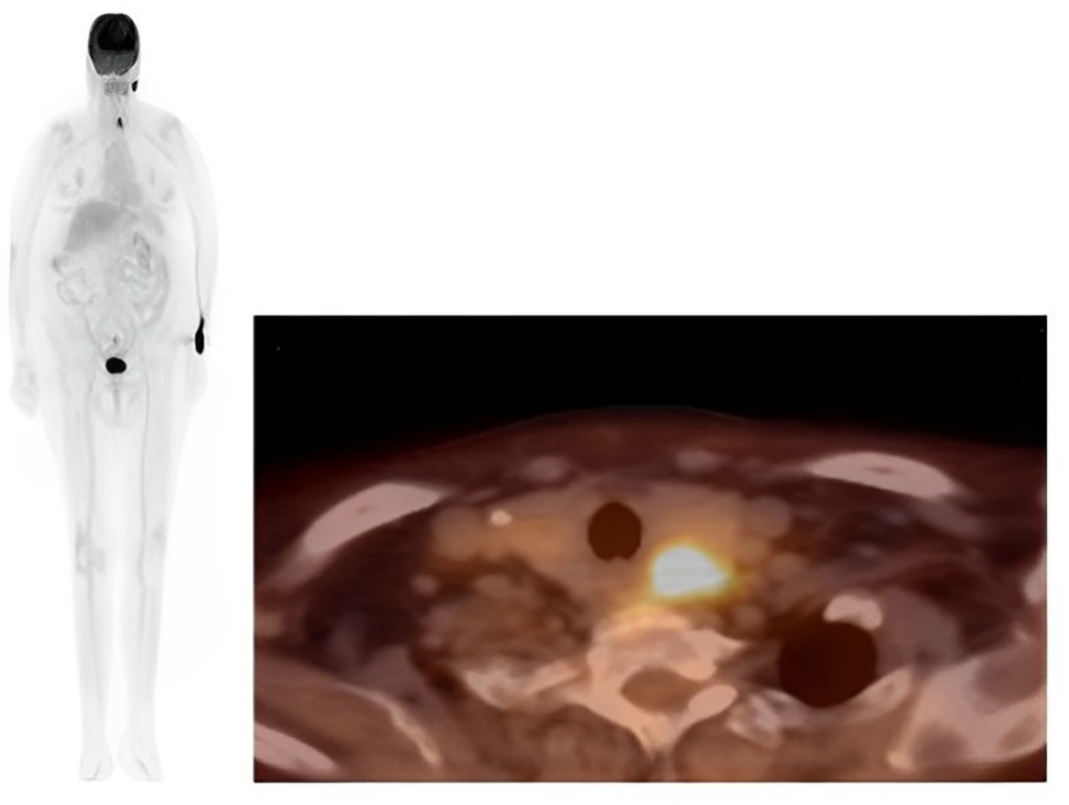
A PET/CT scan of a patient revealing a fused pathological uptake in the thyroid gland, suggesting metastatic involvement in a patient diagnosed with renal cell carcinoma.
